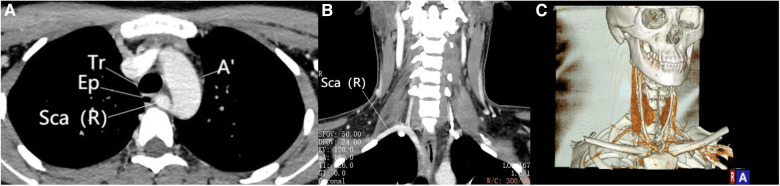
Enhanced CT scan. (A) Viewed in axial view, the right subclavian artery originates from the aortic arch and courses between the vertebrae and the esophagus. (B) Viewed in coronal view, the ectopic course of the right subclavian artery can be observed. (C) The blood vessels under three-dimensional reconstruction can be observed. Sca (R), Right subclavian artery; Ep, Esophagus; Tr, Trachea; A, Aorta.
